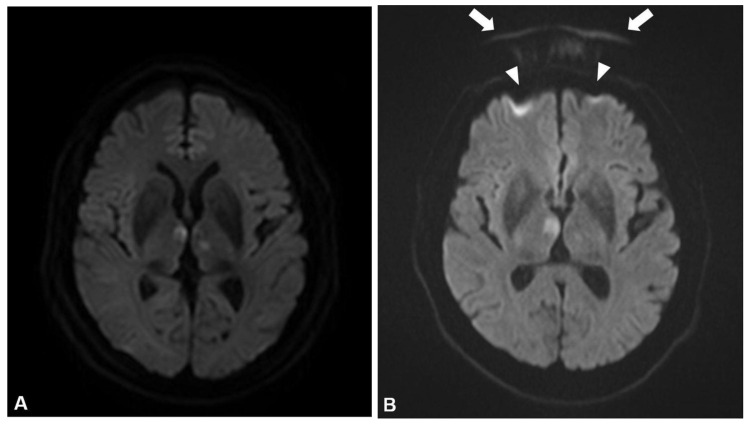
Examples of image quality evaluation of clinical DWI from stroke patients. This patient had a bilateral thalamic acute infarction. (A) A b-1000 image with ideal image quality. The image demonstrated the anatomical structure and tissue of interest without any artifacts. (B) An example of an image of the same patient with a Nyquist ghost artifact and spatial distortion. Nyquist ghost artifact with duplication of the frontal bone in the phase encoding direction (arrows) and mild distortion of both frontal lobes due to a susceptibility artifact (arrowheads). Spatial distortion and the Nyquist ghost artifact were scored as “acceptable” for this image.
